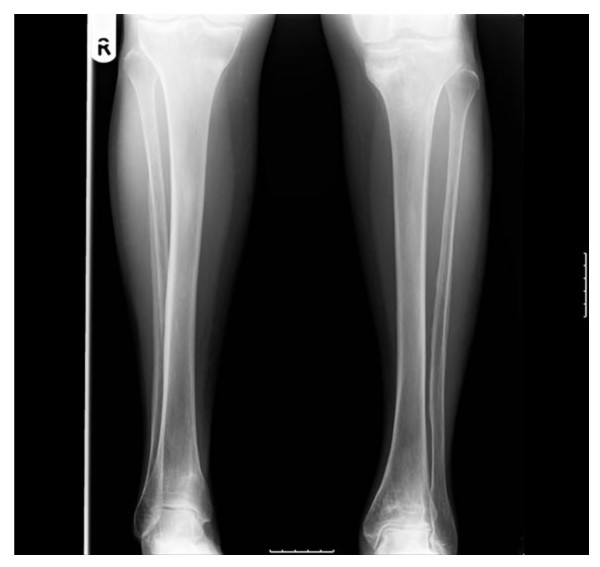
Anteroposterior radiographs of both tibiae six months after diagnosis with resolution of symptoms and healed fractures.
